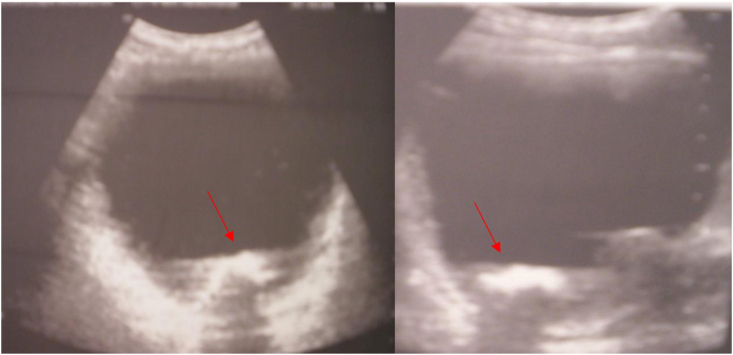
Transabdominal ultrasound of the bladder and prostate: Several hyperechoic formations with posterior shadow cone suggesting prostatic lithiasis.
